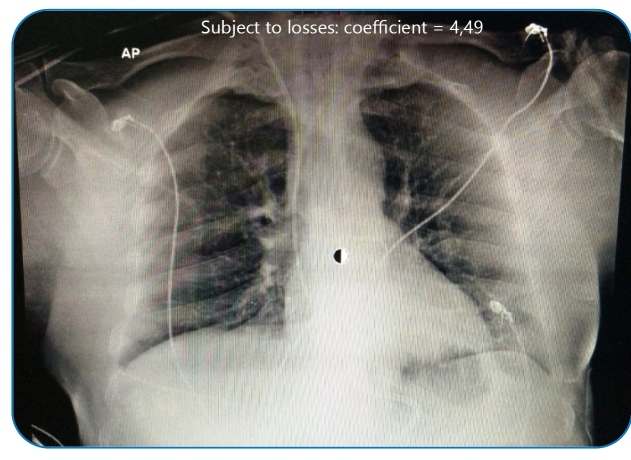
Chest radiography on the 28th day of symptoms.
